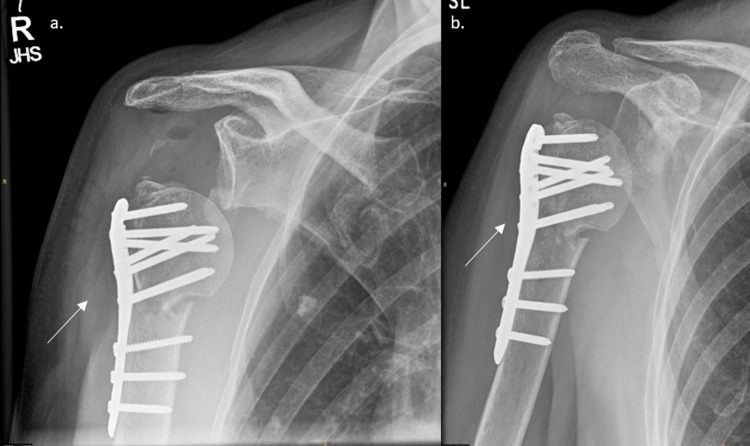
Postoperative radiographs taken at (a) two weeks and (b) six weeks postoperatively showing good fracture union The white arrows indicate the previous fracture.
